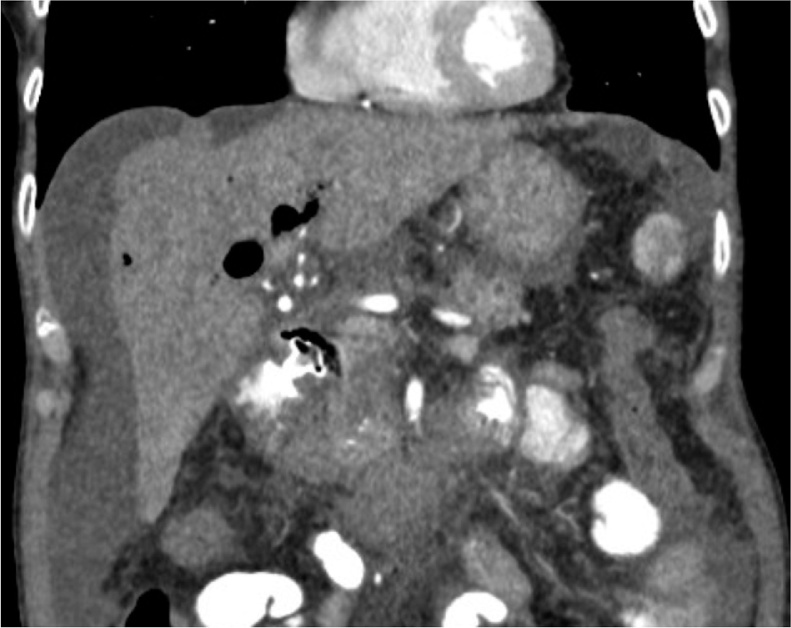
CT scan coronal view shows LAMS in proper position.
